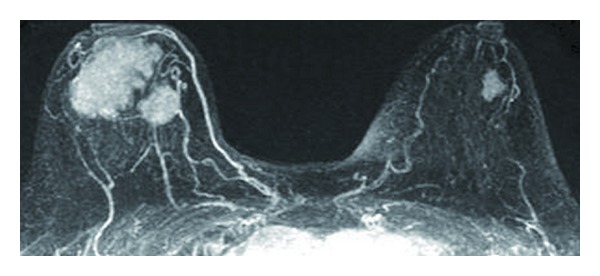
Magnetic resonance imaging of the breast dense microlobulated masses with irregular margins, 43 × 52 × 37 mm and 17 × 23 × 14 mm, were seen in the CDA and A areas of the right breast, respectively. In the C area of the left breast, a similar characteristic mass, 11 × 20 × 15 mm, was also seen.
